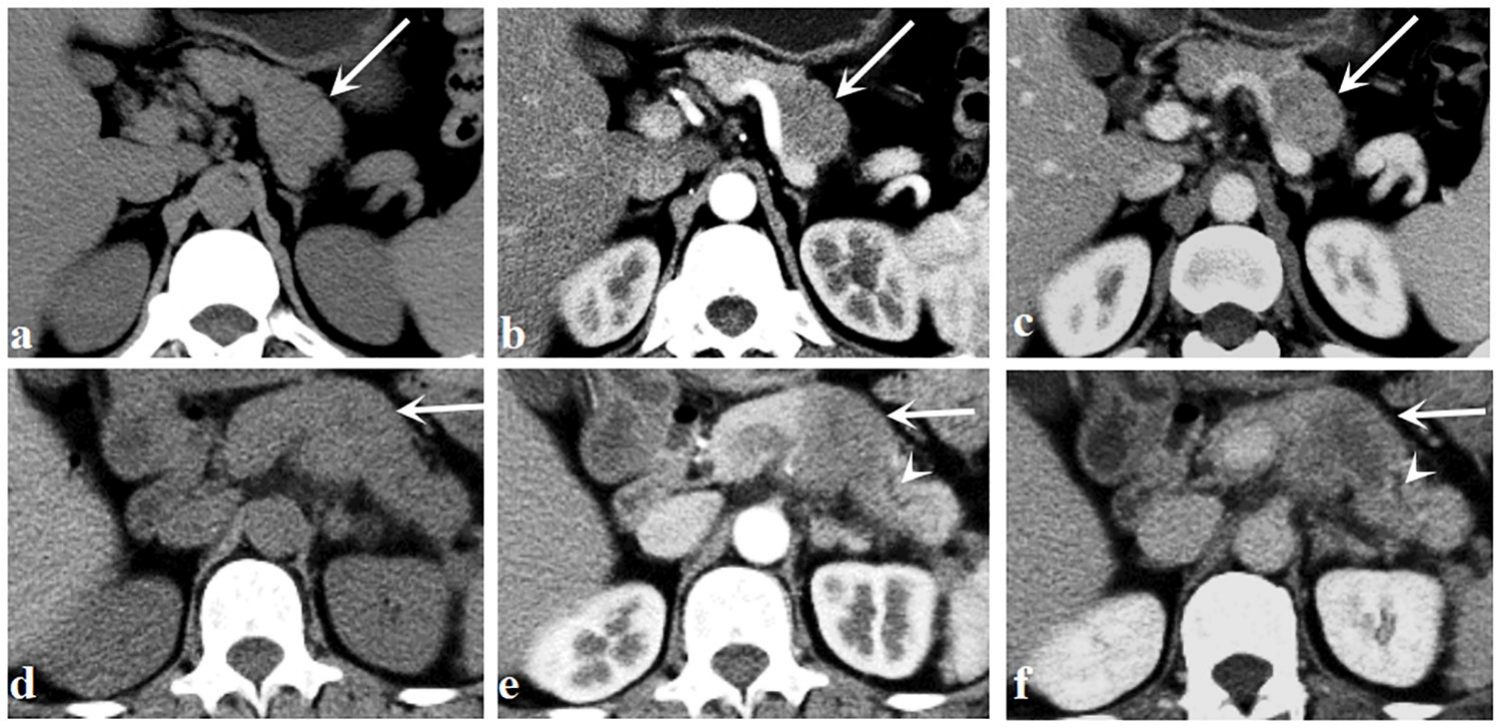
A 42-year-old man with pancreatic neuroendocrine carcinoma. (a—c) Unenhanced (a), arterial (b), and portal venous phase (c) images show a well-defined mass (arrows) in the body of the pancreas. The CT attenuation was 38 HU at the unenhanced phase, 52 HU at the arterial phase, and 75 HU at the portal venous phase. A 60-year-old man with pancreatic ductal adenocarcinoma. (d—f) Unenhanced (d), arterial (e), and portal venous phase (f) images show an ill-defined mass (arrows) in the body of the pancreas. The CT attenuation was 38 HU at the unenhanced phase, 43 HU at the arterial phase, and 55 HU at the portal venous phase. The dialated pancreatic duct is noted (arrowheads).
